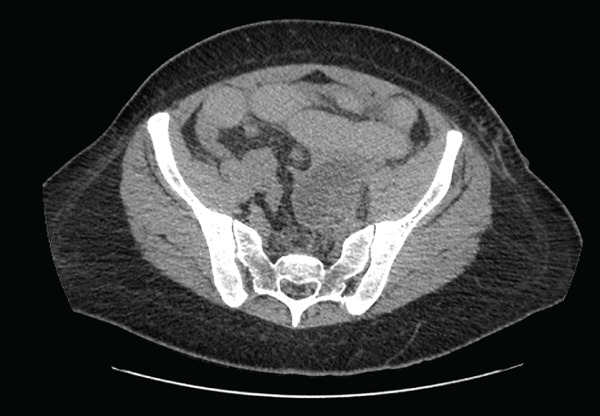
CT image from HOD 3 showing dilated loops of bowel, identified cystic process, and ascites.
